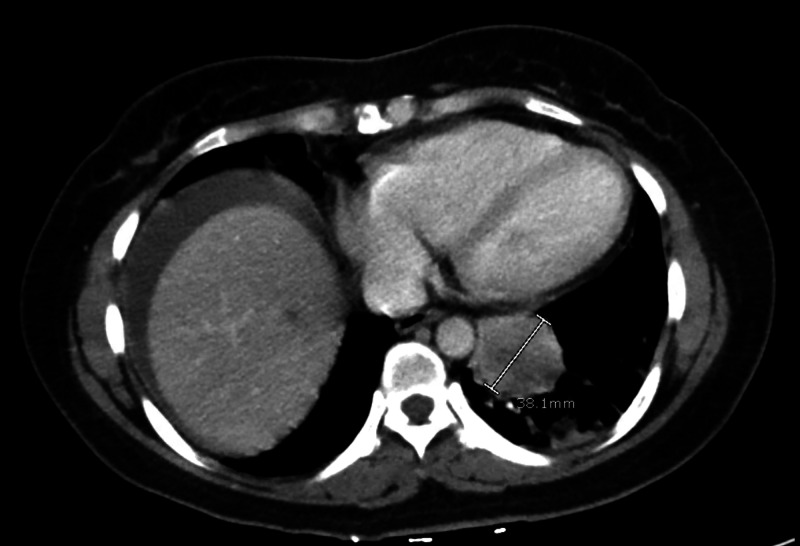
Initial CTAP showing LLL mass-like consolidation measuring 4.1 x 3 cm CTAP: computed tomography of the abdomen and pelvis; LLL: left lower lung; cm: centimeter
